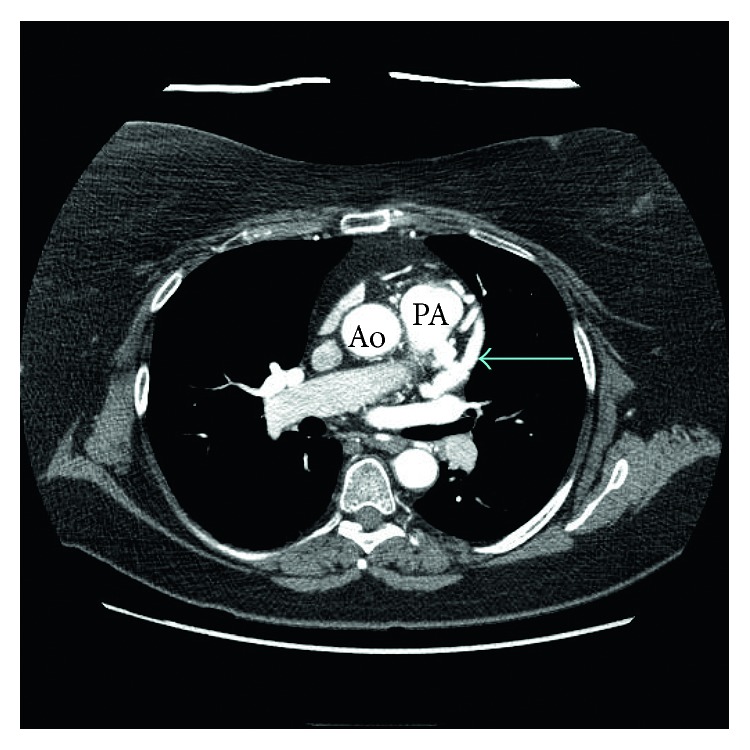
Axial view of multidetector cardiac CT angiogram shows the origin of the left main coronary artery (LMCA) from the main pulmonary artery (blue arrow).
